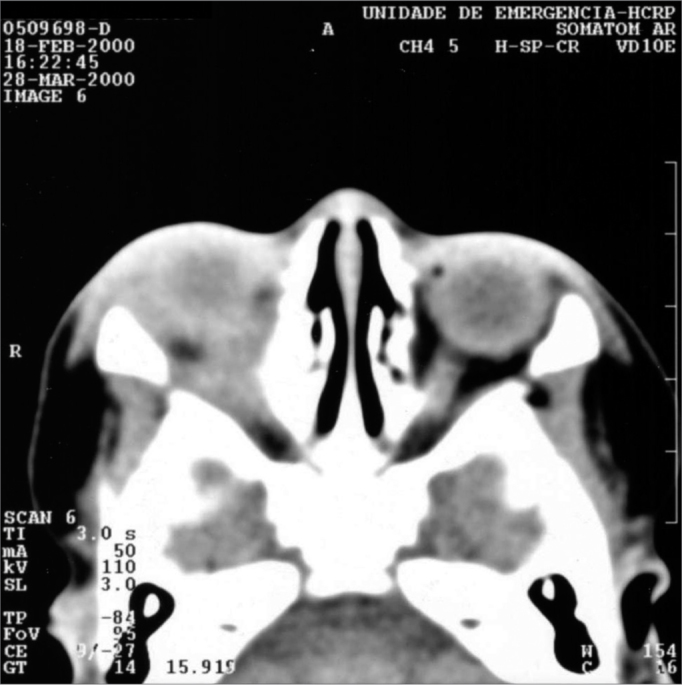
Axial view CT scan of the orbit showing orbitary abscess on the right side.
